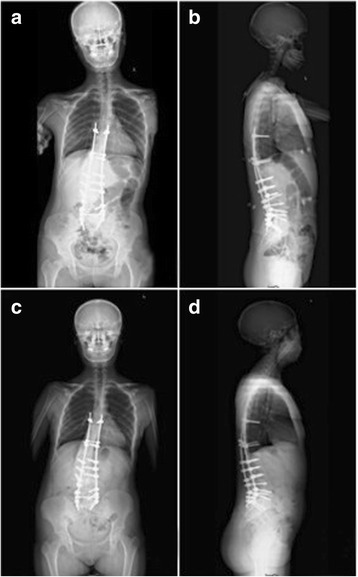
Postoperative anterioposterior radiographic image (a) showed Cobb’s angel decreased to 29 ° and distant from the mid-point of superior border of sacrum to the C7 plumb line reduced to 3.7 cm, indicating reconstruction of coronal balance. Later radiographic image (b) revealed the correction of thoracolumbar kyphosis and sagittal balance (1.9 cm). A 6-month follow-up radiographic image showed no aggravate of scoliosis or spinal imbalance (c and d)
